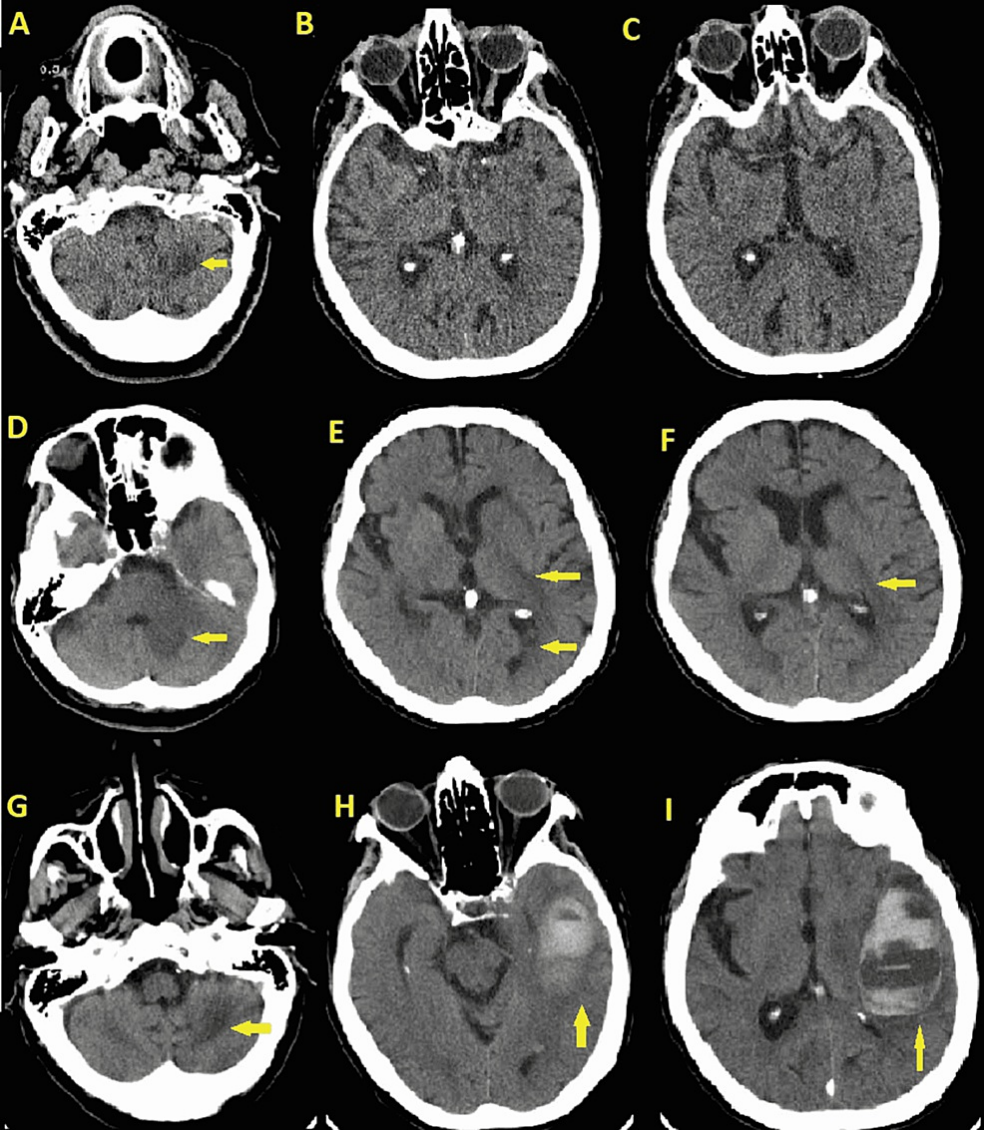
Computed tomography (CT) scan of the brain with/without contrast (A-C) CT brain stroke code on admission showing an old left cerebellar infarct (arrow in A). There is no evidence of acute infarct, hemorrhage, or extra-axial collection. (D-F) CT after 12 hours from admission showing an increasing hypodensity in the left temporal lobe (arrow in E, F) as well as within the left cerebellar hemisphere (arrow in D) consistent with evolving relatively acute infarcts. While there is no hemorrhage within these areas of evolving infarction although there is a small amount of blood within the left occipital horn (arrow in E). There is no developing midline shift although there is a mass effect on the fourth ventricle as a result of the evolving left cerebellar infarct. (G-I) Last CT before expiration showing a new temporal lobe intraparenchymal hematoma which measures approximately 5.9 x 3.8 cm (arrow in H, I) which results in mass effect on the left lateral ventricle and a resultant left to right midline shift of 5 mm. Small amount of blood within the temporal horn of the left lateral ventricle is consistent with intraventricular extension. No extra-axial collection identified. Hypodensity within the left cerebellar hemisphere (arrow in G) is similar to that of previous CT.
